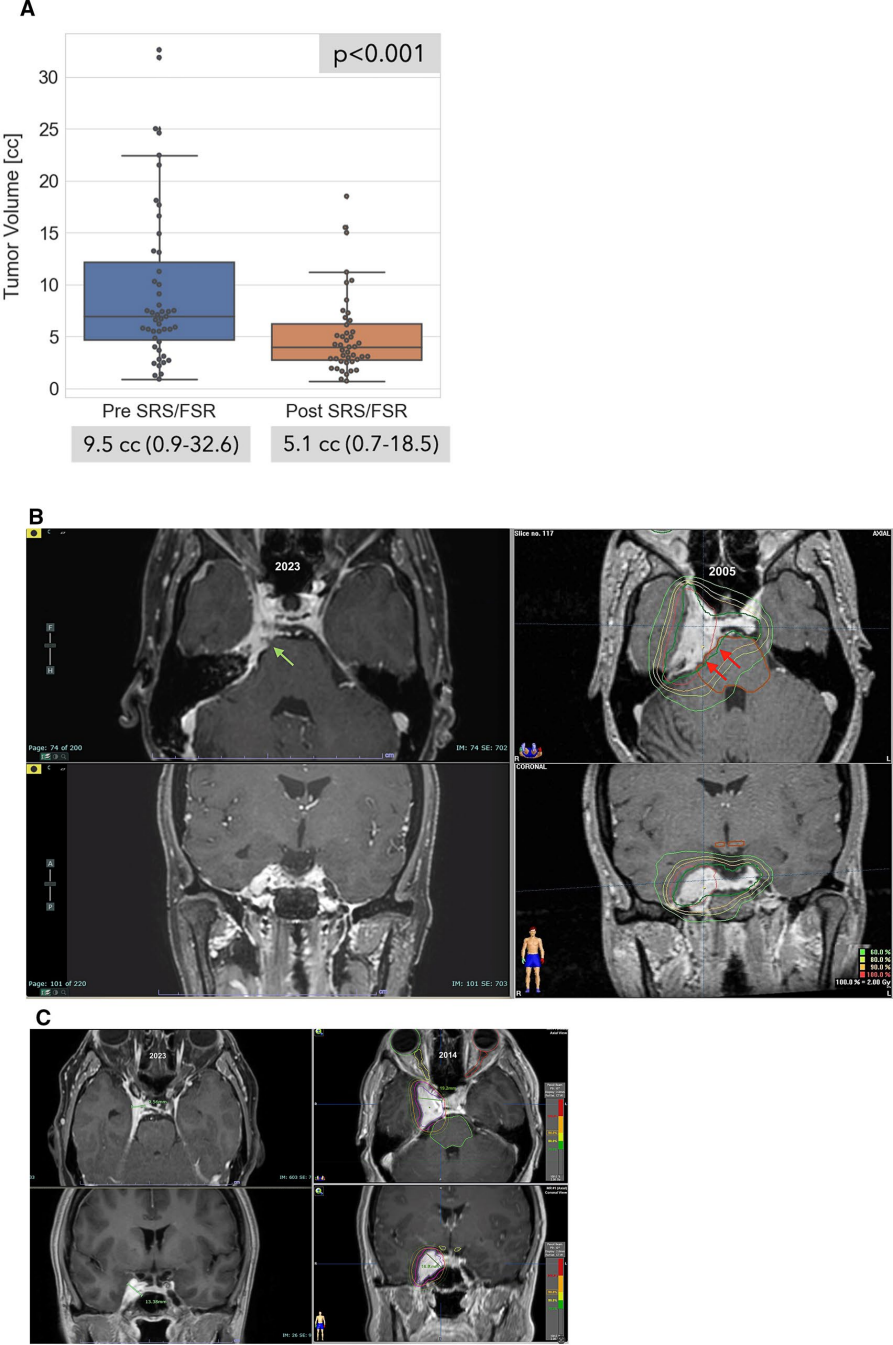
Radiological Tumor Response. Graphic depiction of mean pre- and post-SRS/FSR tumor volumes (A). As demonstrated in the graph, the mean pre-SRS/FSR treatment tumor volume was 9.5 cc compared to the mean post-treatment tumor volume which was 5.1 cc. A 100% tumor control rate was therefore identified. A representative case of a patient with CSM who was treated in the year 2005. A significant tumor reduction was detected at the last follow-up MRI in 2023. The red and green arrows represent the tumor interface with the brainstem, which is markedly reduced (B). Another representative case of a CSM patient who was treated in the year 2014 and a follow-up scan that was conducted in 2023. The bar within the CSM mass boundaries represents the calculated tumor diameter and the difference detected between the two time points. Tumor volume reduction was 57%. The figures also contain the radiation dose distribution and treatment isodose lines as well as the optic apparatus and brainstem markings (C)
